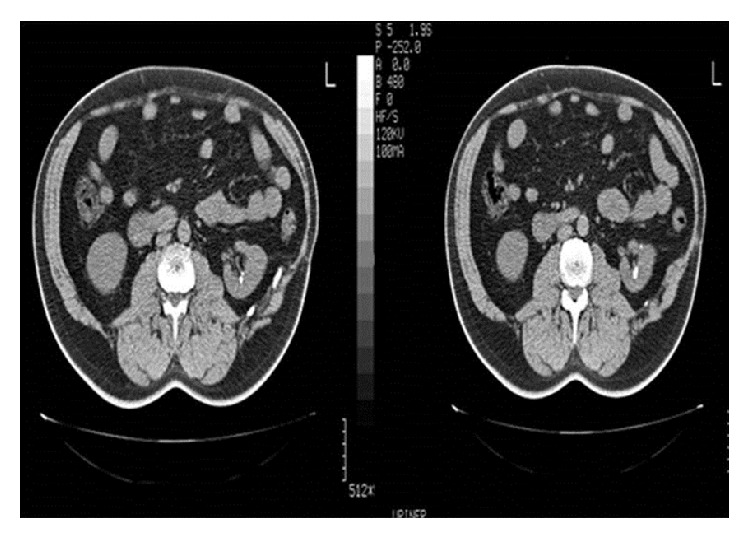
Preoperative CT imaging showing left kidney stone.
